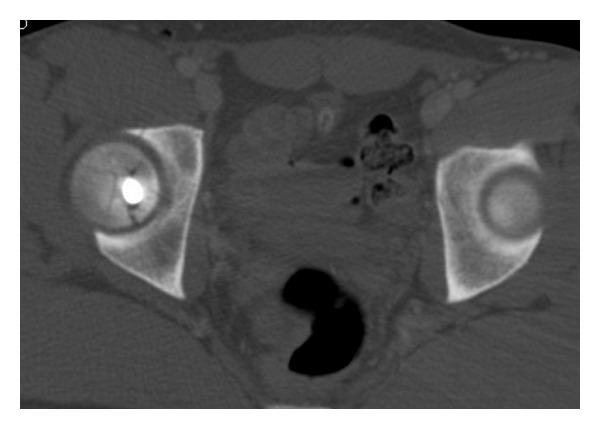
Axial CT scan of the pelvis shows the location of the bullet in the femoral head with accompanying fracture.
